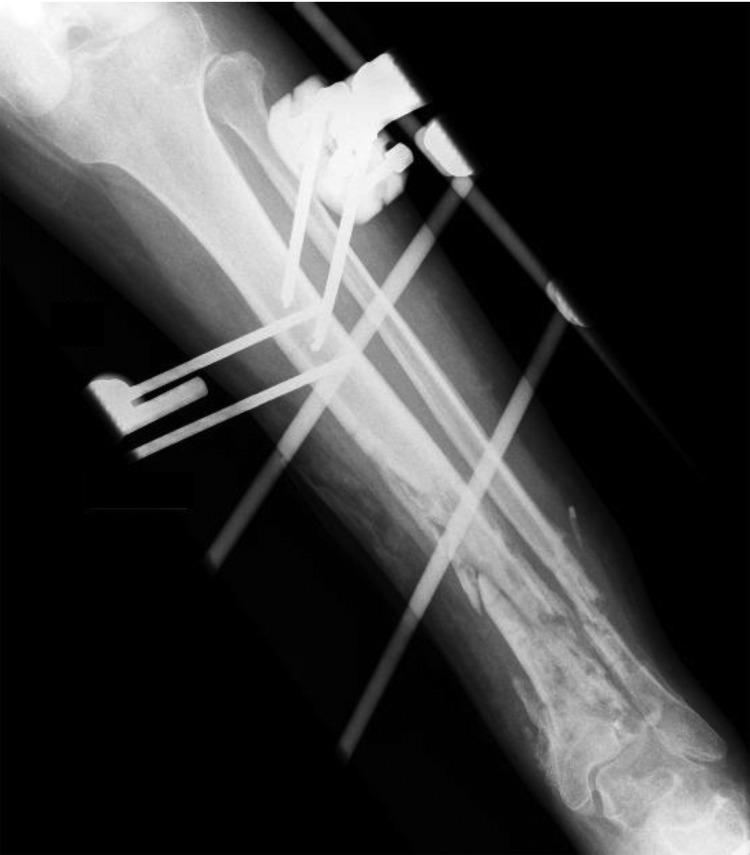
Radiograph of left leg of patient with *Clostridium tetani* infection, showing delayed bone consolidation 11 months after fracture.
